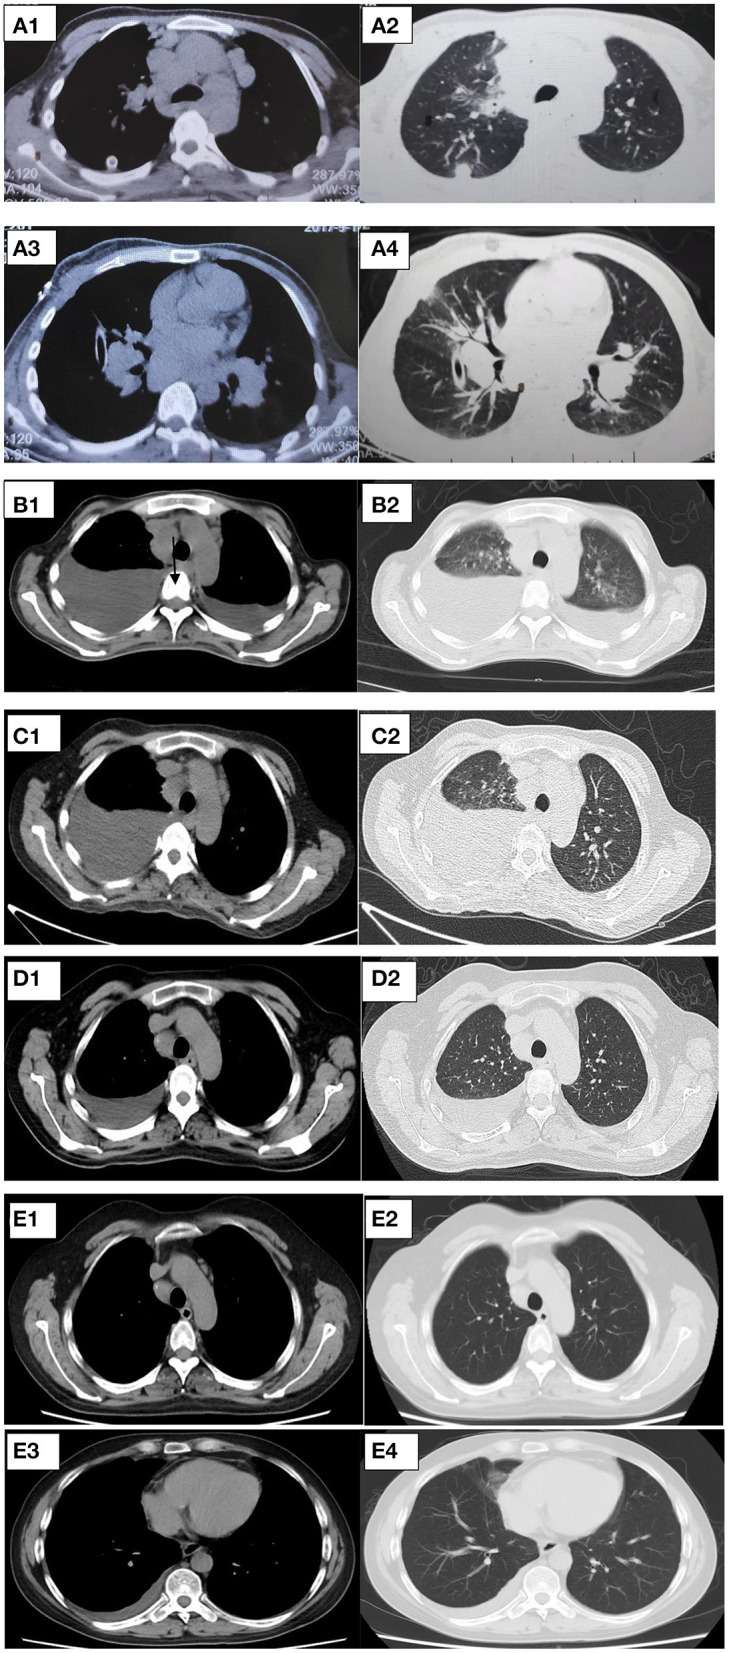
(A–D) Representative CT cuts of the thorax during the course of the disease. (A) Chest CT in 2017. (B) Pre-glucocorticoid therapy (September 29, 2020, chest CT). (C) One month after glucocorticoid therapy (November 25, 2020, HRCT). (D) Six months after glucocorticoid therapy (March 20, 2021, HRCT). (E) Nine months after glucocorticoid therapy (June 30, 2021, chest contrast-enhanced CT). CT, computed tomography; HRCT, high resolution computed tomography.
